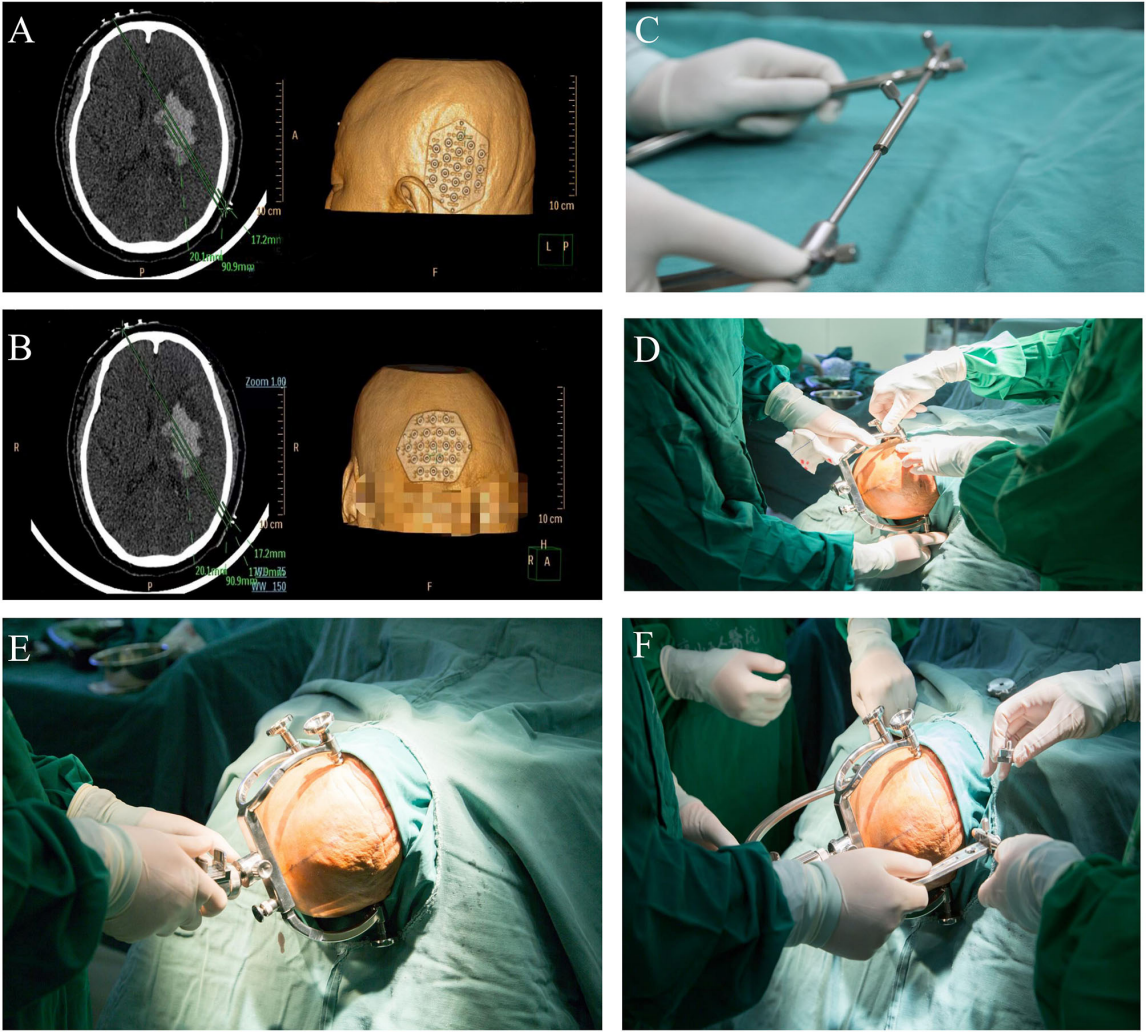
(A,B) show that the accurate puncture site, reference point, puncture route, puncture depth, and drill depth are located on the skin by performing a CT scan with 1 mm for each layer using the PISP (Philips IntelliSearch Portal v5.0.2.10010) system. The brain surgery head frame is correct in (C), and (D–F) is the fixation process of the head frame.
